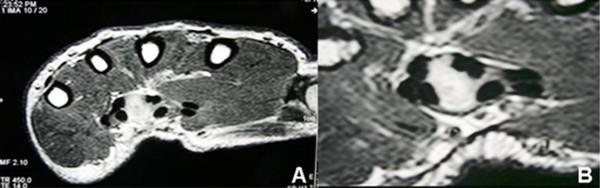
A. MRI (T1-weighted image) of the left hand showing a soft tissue mass into the carpal tunnel B. Under higher magnification, the tumor looks homogenous, well-defined and it is surrounded by the flexor tendons.
